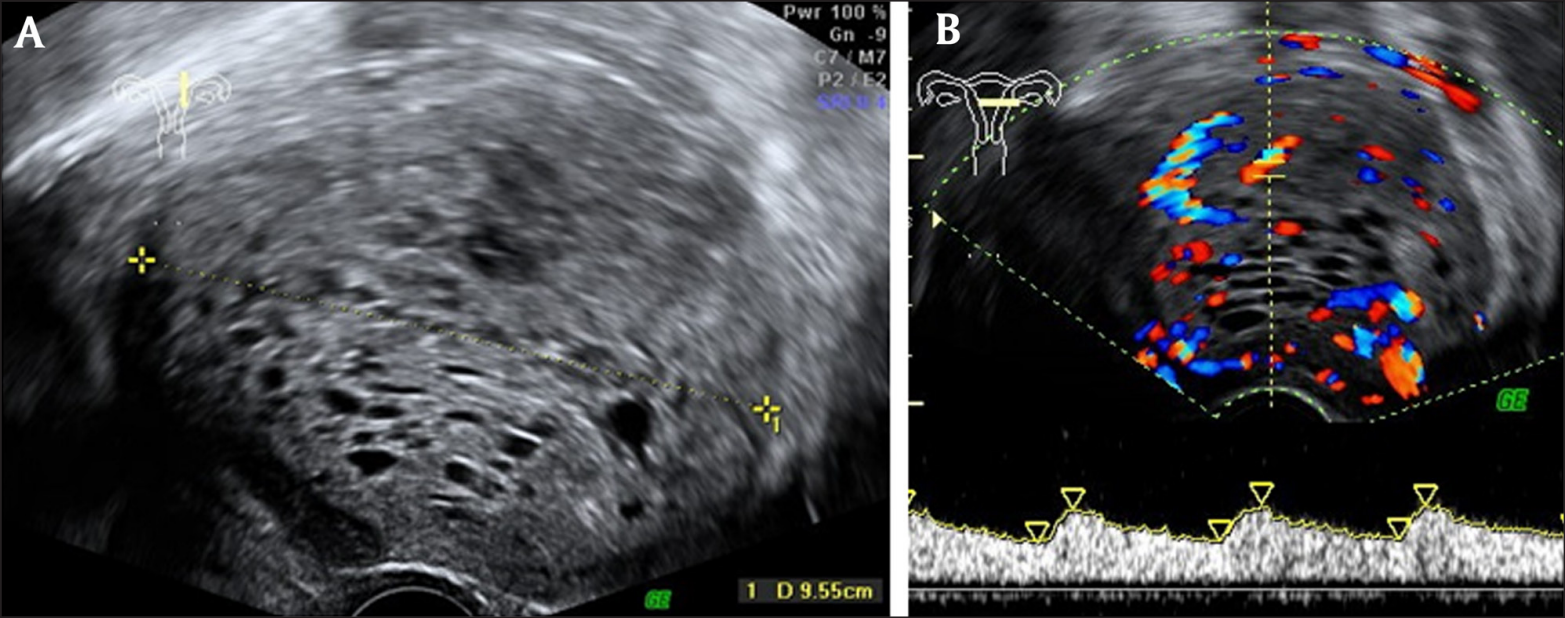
(A) Endovaginal ultrasound: the nearly 10 cm multicystic mass between the yellow crosses. (B) Color Doppler endovaginal ultrasound: the tumor appears hypervascular with low resistance arterial spectra (yellow arrows).
